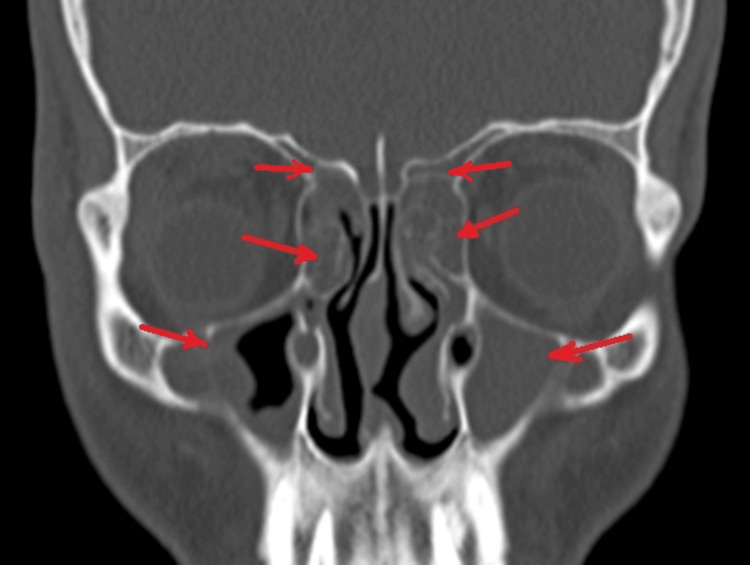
Contrast-enhanced CT showing collection in bilateral maxillary, ethmoidal, and frontal sinuses (red arrows).
